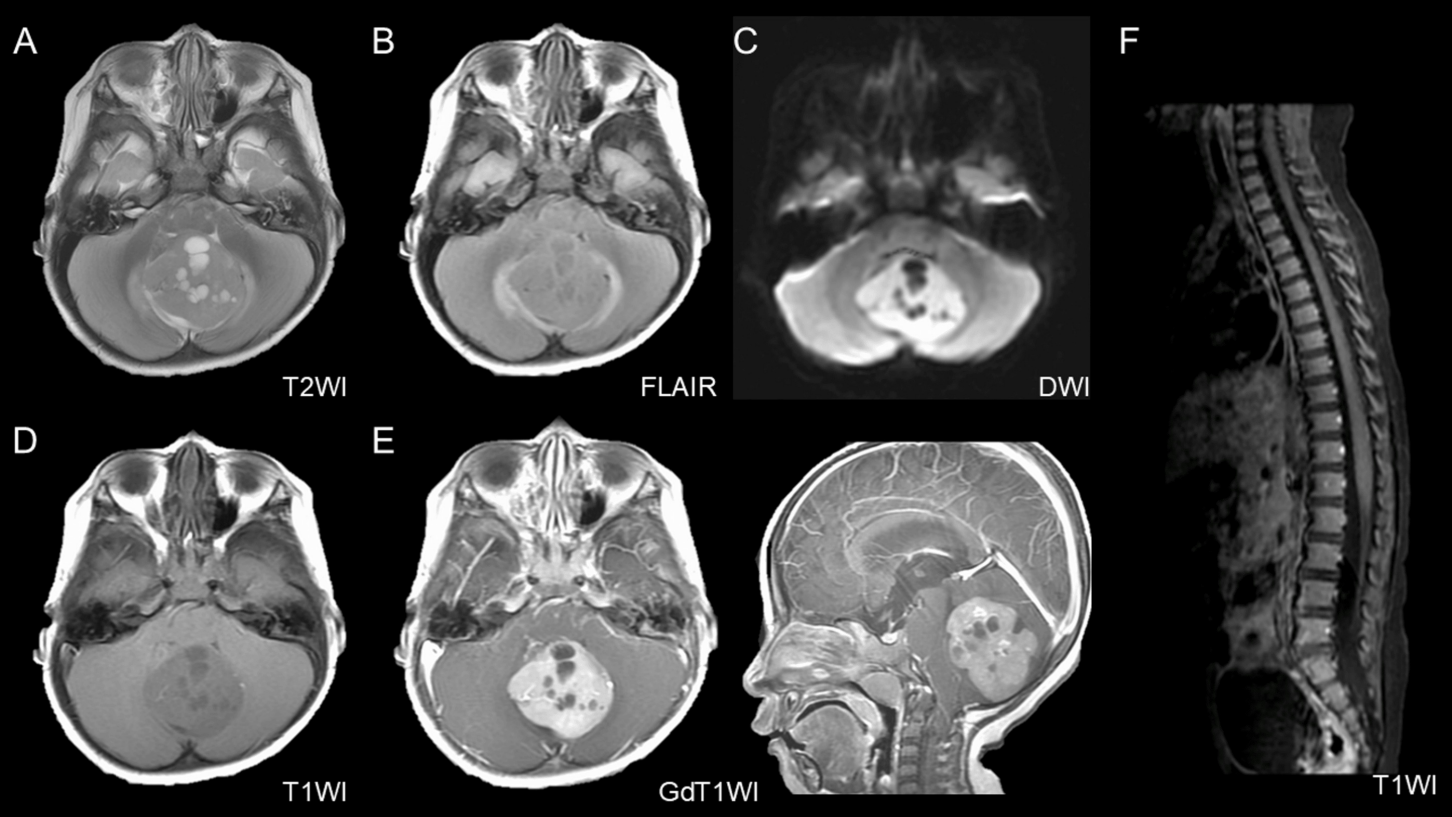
Preoperative brain MRI showing a posterior fossa tumor. A 45-mm tumor was observed in the fourth ventricle. The tumor shows multiple cystic components on T2-weighted imaging (T2WI) (A) and peritumoral edema on fluid-attenuated inversion recovery imaging (FLAIR) (B). It appears hyperintense on diffusion-weighted imaging (DWI) (C). On plane T1-weighted imaging (T1WI) (D), the tumor appears slightly hypointense and exhibits homogeneous enhancement after gadolinium administration (E). T1-weighted imaging showed no evidence of spinal dissemination (F).
